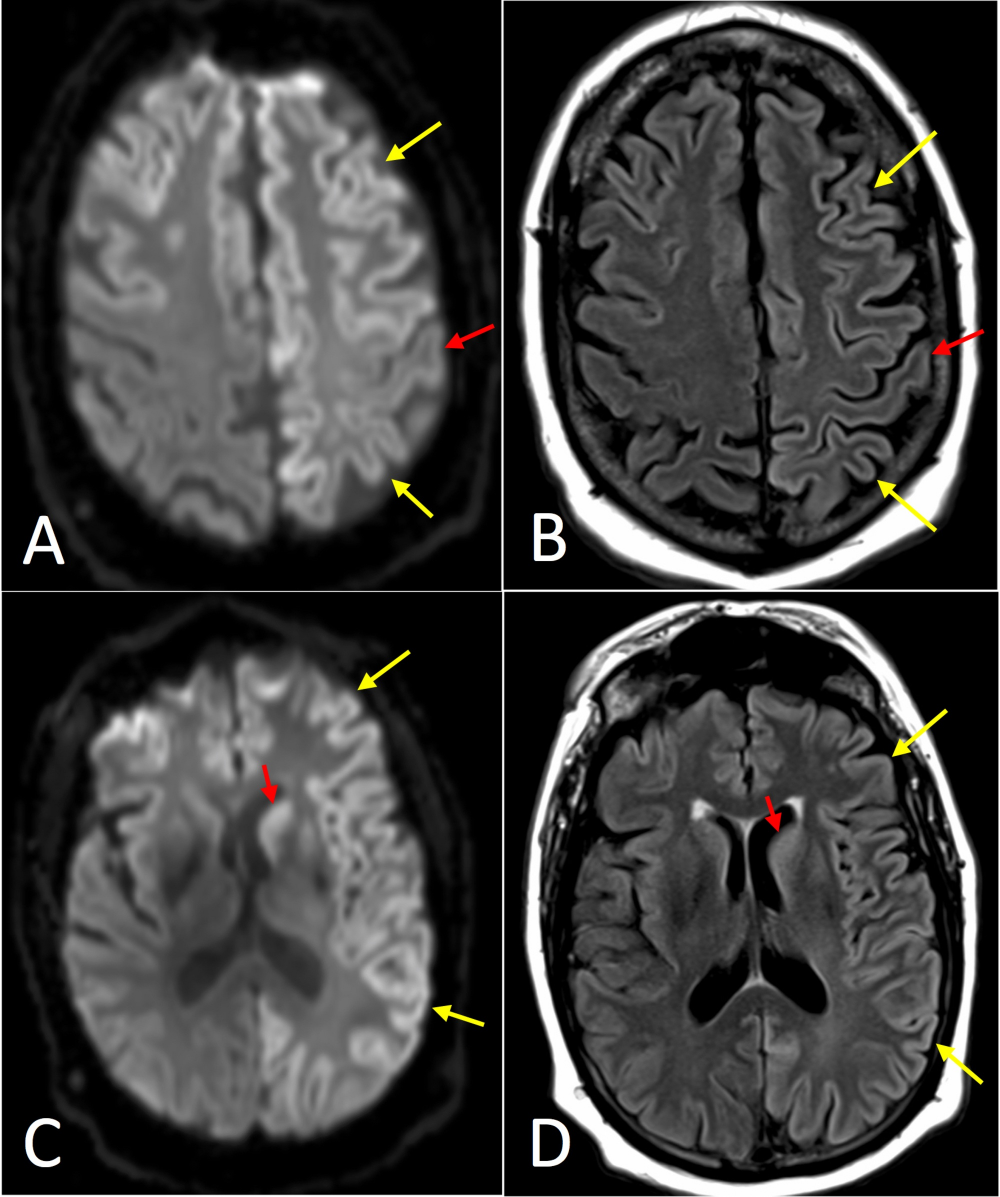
Axial magnetic resonance imaging (MRI) of Case 5. (A) Diffusion-weighted image (DWI) at the level of the centrum semiovale demonstrating asymmetrically increased signal in the frontal and parietal cortex (yellow arrows) on the left with relatively decreased signal in the precentral gyrus (red arrow). (B) T2-weighted fluid attenuation inversion recovery (T2-FLAIR) image with less corresponding hyperintense signal. (C) DWI at the level of the basal ganglia demonstrating asymmetrically increased signal in the neocortex (yellow arrow) and striatum (red arrow). (D) T2-FLAIR image with less corresponding hyperintense signal.
